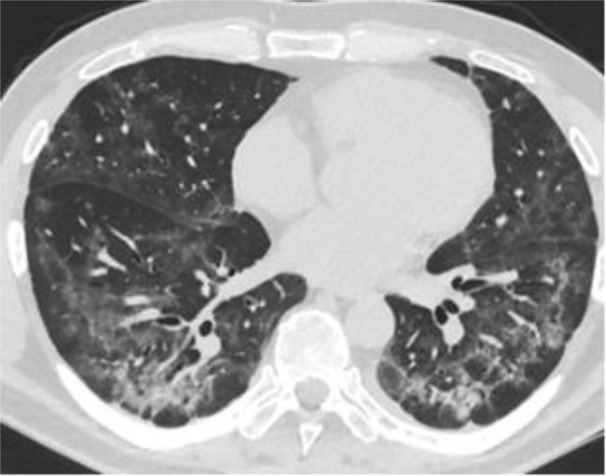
Chest MS CT scans after a course of inhalation with Xe/O_2_ gas mixture
